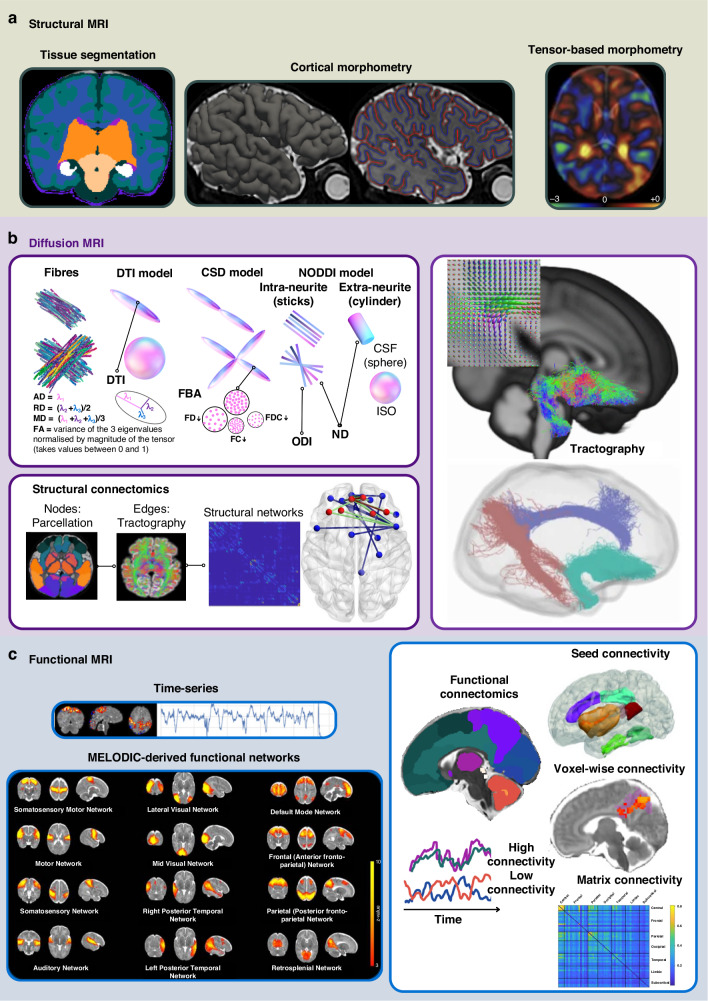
Advanced neuroimaging approaches. a Structural MRI: Left and Center, Brain segmentation and cortical morphometry of a neonate from dHCP data release 3^44^; Right, Tensor-based morphometry t-statistic map adapted from Lautarescu and colleagues.^57^
